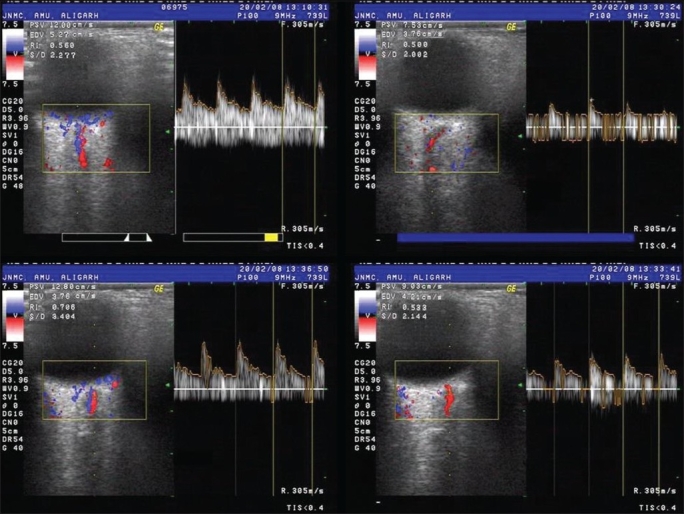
Clockwise from top left: Color Doppler images of central retinal artery blood flow at baseline and at 3, 6 and 9 min after chilling
